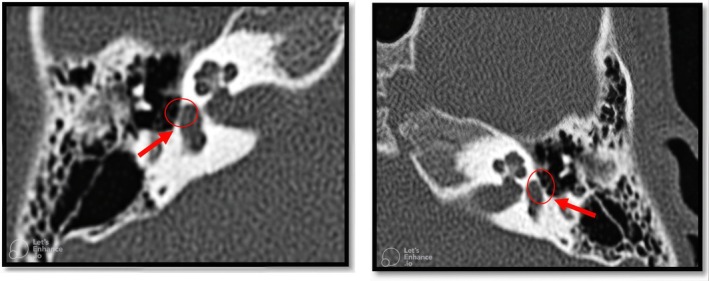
The CT scan images. The location of the footplate is marked in both images from different angles, which is a little thicker in these pictures that increase the footplate fixation probability.
